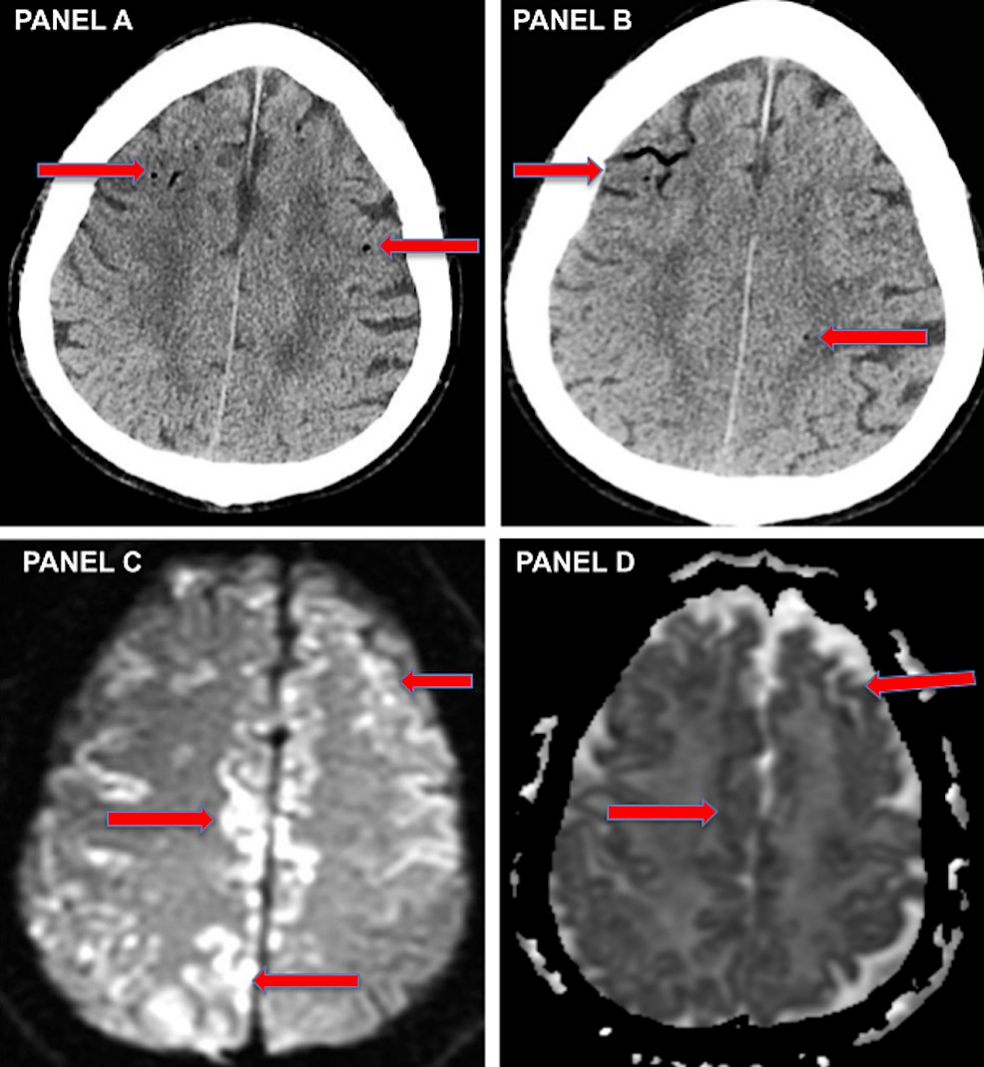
CT head non-contrast and MRI brain without contrast images (A,B) CT head non-contrast: axial cuts showing multiple foci of air in bilateral cerebral arteries in both hemispheres. (C, D) MRI brain DWI and ADC sequence axial cuts showing diffuse areas of restricted diffusion with bilateral gray matter involvement and cortical ribboning pattern.
